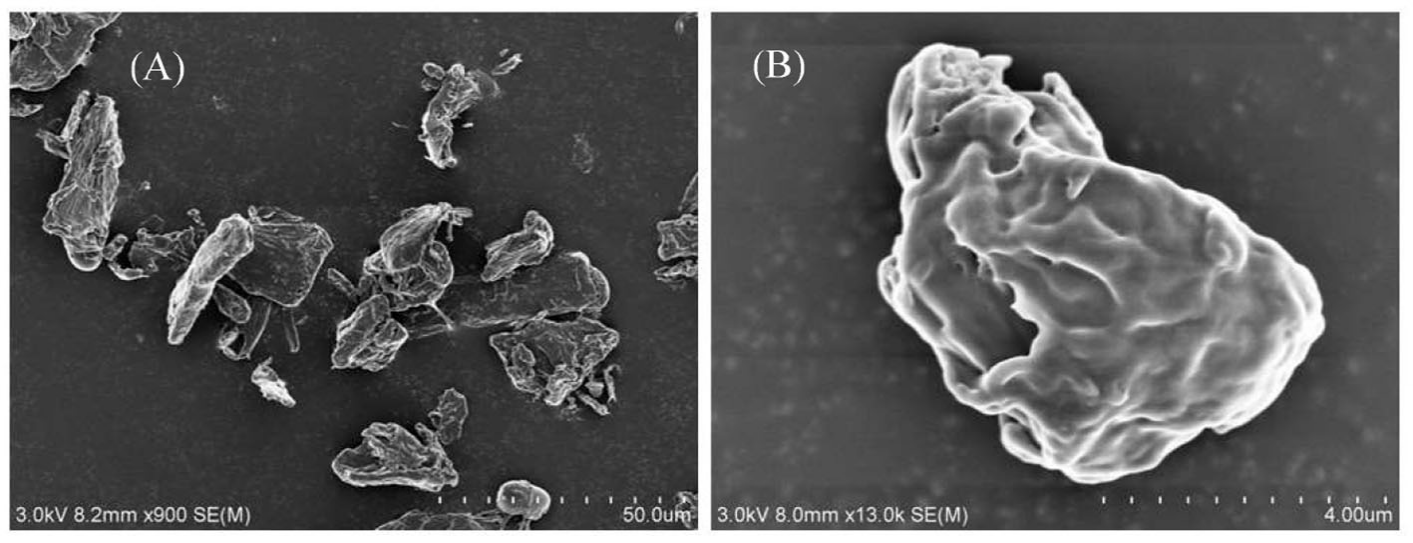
SEM of cellulose particles as powders (A) and was ultrasonically dispersed in water (0.3%) (B).
